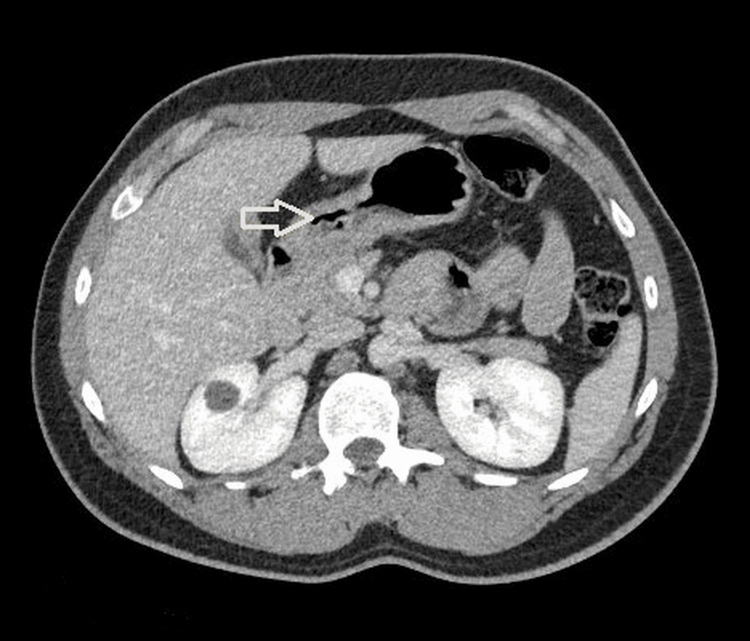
Axial contrast-enhanced CT of the abdomen demonstrating a well-circumscribed low-attenuation cystic lesion adjacent to the gallbladder (arrow), initially interpreted radiologically as a probable gallbladder diverticulum
